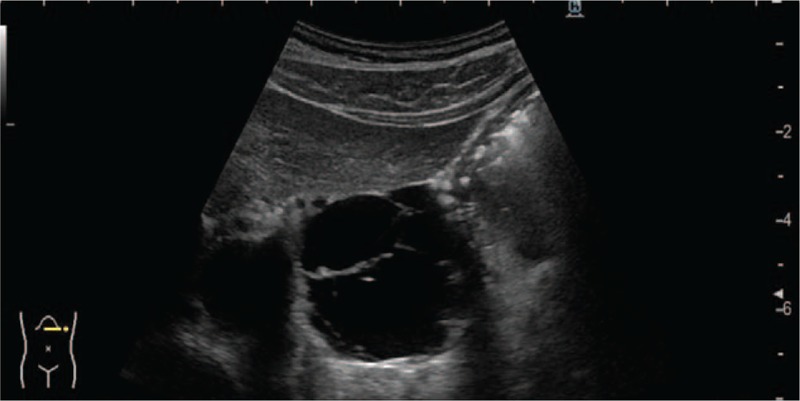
Abdominal ultrasonography findings. Multilocular cystic lesion is evident in lesser curvature of stomach.
